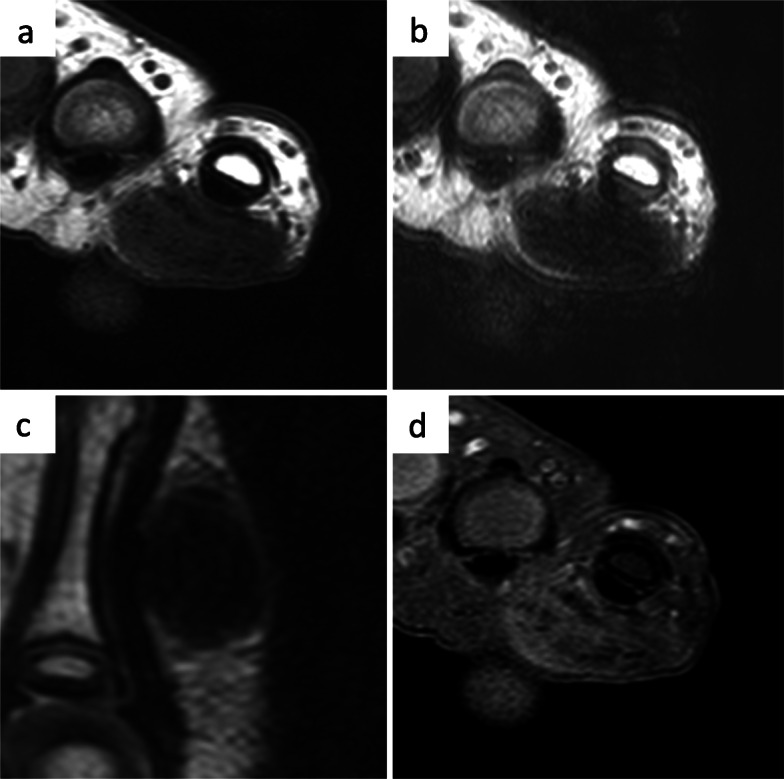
Preoperative magnetic resonance images. The mass shows a iso signal intensity to muscle on T1-weighted axial images and b, c homogenous and low signal intensity to muscle on T2-weighted axial and sagittal images, respectively. d The mass is peripherally enhanced in T1-weighted fat saturation axial images with gadolinium enhancement
